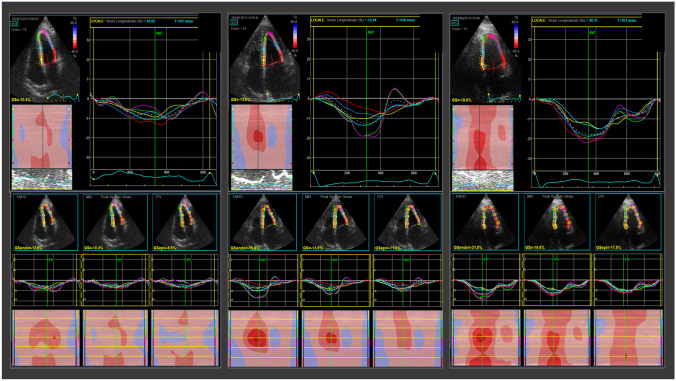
Left ventricular global longitudinal strain and cardiac allograft vasculopathy. This picture shows left ventricular longitudinal strain and three-layer specific longitudinal strain in three different heart transplant patients, with grade 3 cardiac allograft vasculopathy (CAV), with grade 1 CAV and without CAV, from left to right respectively. Left ventricular longitudinal strain assessed in apical 4-chamber view appears significantly reduced in presence of diffuse CAV, whereas it is almost comparable to normal subjects in the absence of this complication
